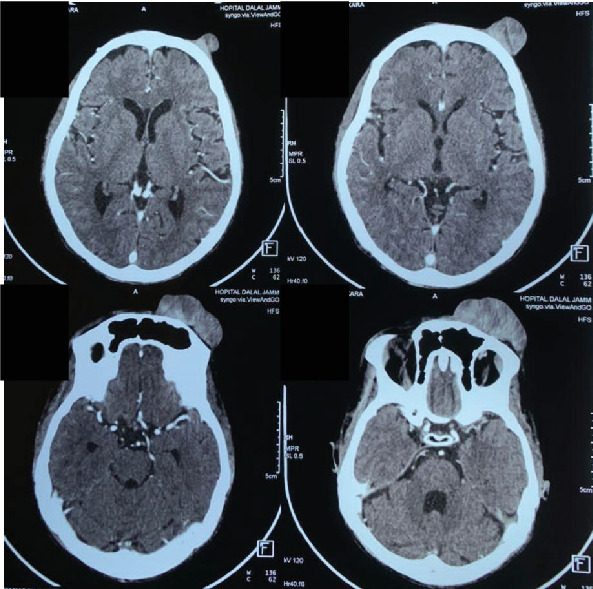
Contrast-enhanced head CT (axial view) showing no evidence of brain involvement by the tumor.
